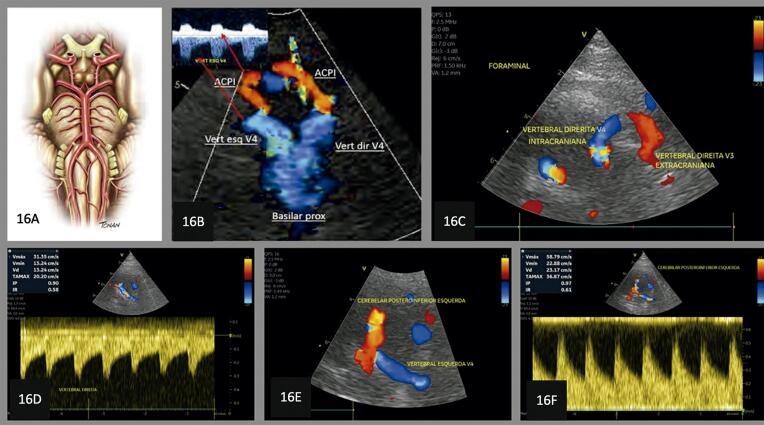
Anatomia de segmentos intracranianos de vertebrais, basilar e ramos (16A); janela foraminal: mapeamento em cores de fluxo em luzes de vertebrais (V4) e ramos cerebelares posteroinferiores (ACPI – 16B); mapeamento em cores de transição de vertebral V3 (extra) para V4 (intracraniana – 16C); curva espectral de Doppler pulsátil afastando-se do transdutor (V4) (16D); mapeamento em cores de fluxo em ramo cerebelar posteroinferior esquerdo (fluxo em direção ao transdutor – 16E); e curvas espectrais de Doppler pulsátil em ACPI esquerda (16F). ACPI: ramo cerebelar posterior inferior.
